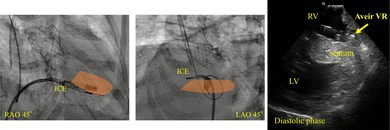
Leadless pacemaker (LP) plays an important role in the management of brady‐arrhythmias, particularly in patients who are at high risk of CIED infection. LPs are characterized by a high procedural success rate and a low incidence of complications with up to 50% reduction compared with a conventional transvenous pacemaker (TVPM) [1, 2]. However, cardiac perforation remains one of the most serious complications. It often requires surgical intervention, leading to be life‐threatening [3]. For this reason, delineation of right ventricular (RV) anatomy using contrast agents is important during implantation. However, the management of LP implantation in patients with severe contrast allergy or renal dysfunction who are high risk for contrast exposure has recently become an important clinical issue. Here, we report a case in which intracardiac echocardiography (ICE) was utilized to visualize RV anatomy and enable the safe implantation of LP in a patient with a severe contrast allergy.
